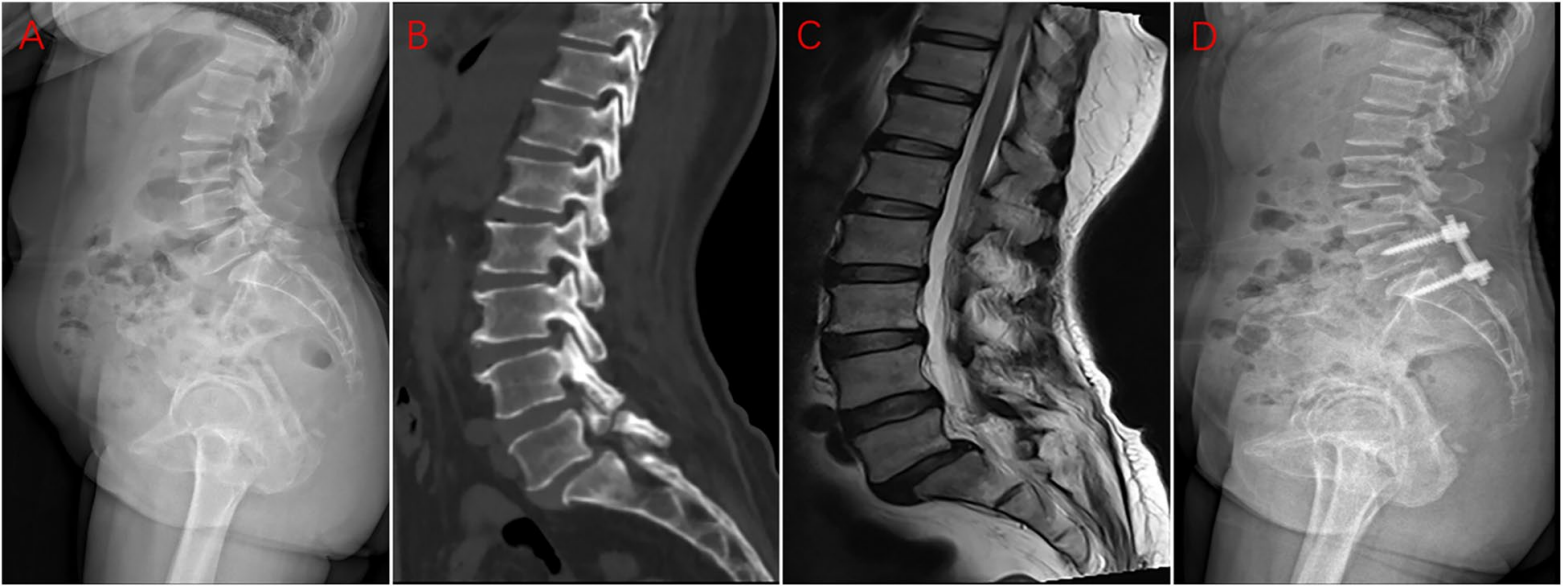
The preoperative lateral X-ray image (a), preoperative sagittal CT image (b), preoperative sagittal MRI image (c), and postoperative (d) lateral X-ray image of a 60-year-old female patient, who underwent L5-S1 PLIF, with 4 screws to correct lumbar spondylolisthesis
